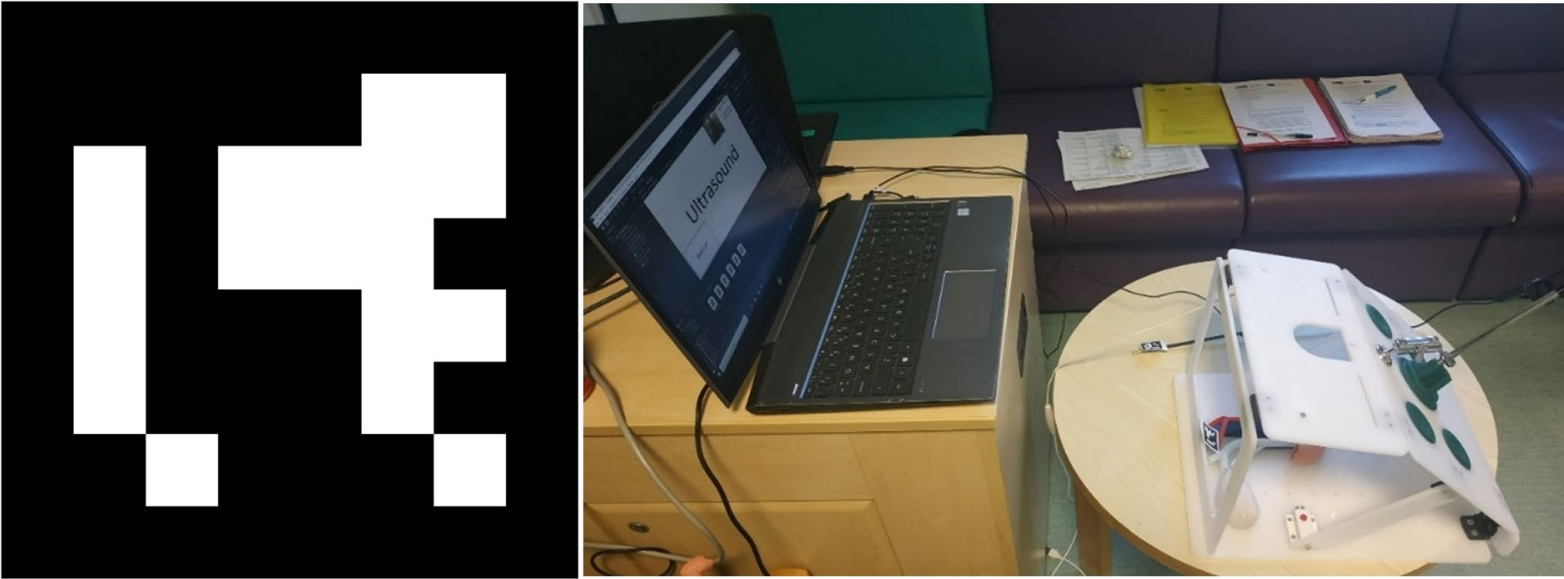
ArUco marker (left); setup for the augmented reality ultrasounds training (right)
